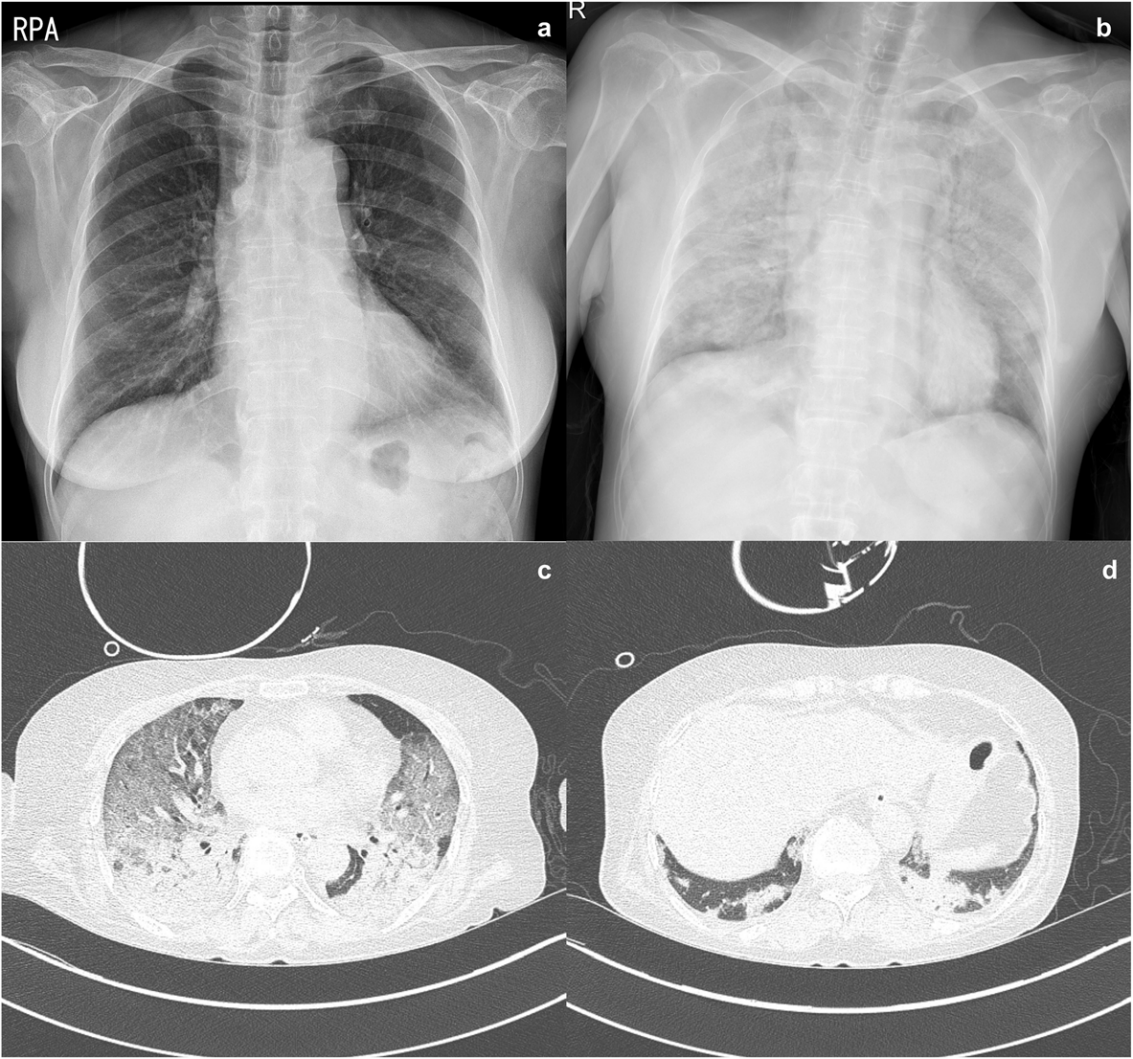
Chest radiographic images before and at the presentation of acute respiratory failure. Chest postero-anterior radiography taken about one month after initiating rituximab therapy shows no clear haziness or infiltration in both lung fields (a). At the presentation of acute respiratory failure with altered mentality (in the sixth week after starting rituximab) there was diffuse bilateral infiltration in both lung fields with relative sparing of both lower lung fields (b). Axial high-resolution computed tomography images show diffuse ground glass opacity in both upper lungs with consolidation in dependent areas (c); The lung base is relatively spared (d)
